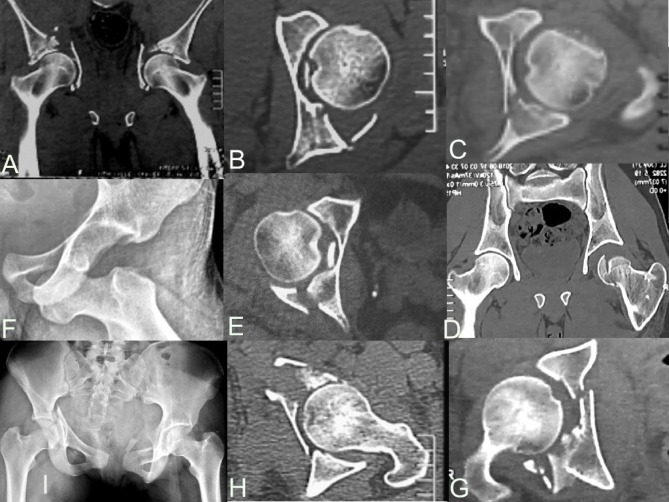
CT scans and plane radiographs showing various radiological modifiers: (A) roof impaction, (B) Marginal impaction, (C) femoral head impaction, (D) head fracture, (E) intra-articular fragment, (F) preoperative dislocation, (G) fracture site comminution, (H) Quadrilateral plate involvement, (I) pelvic ring involvement.
